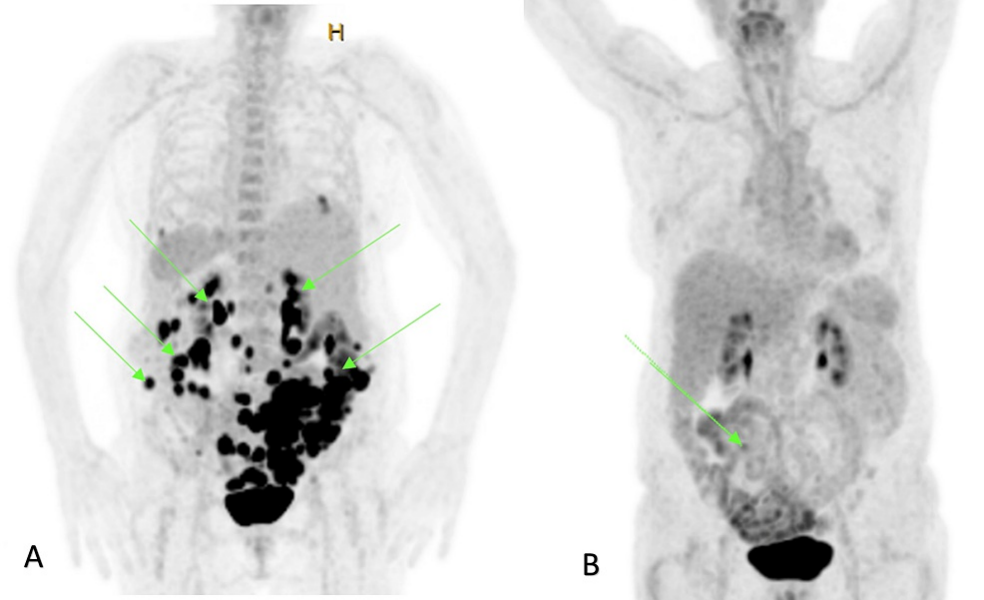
PET/CT before (A) and after (B) neoadjuvant chemotherapy showing near complete response. Arrows indicate PET-avid lesions.
